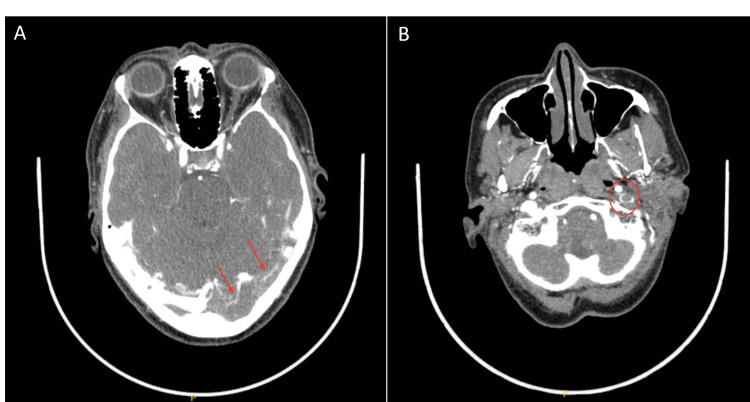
CT angiogram demonstrating a filling defect throughout the left transverse sinus and left sigmoid sinus (A) extending below the jugular bulb into the superior aspect of the jugular vein (B).
